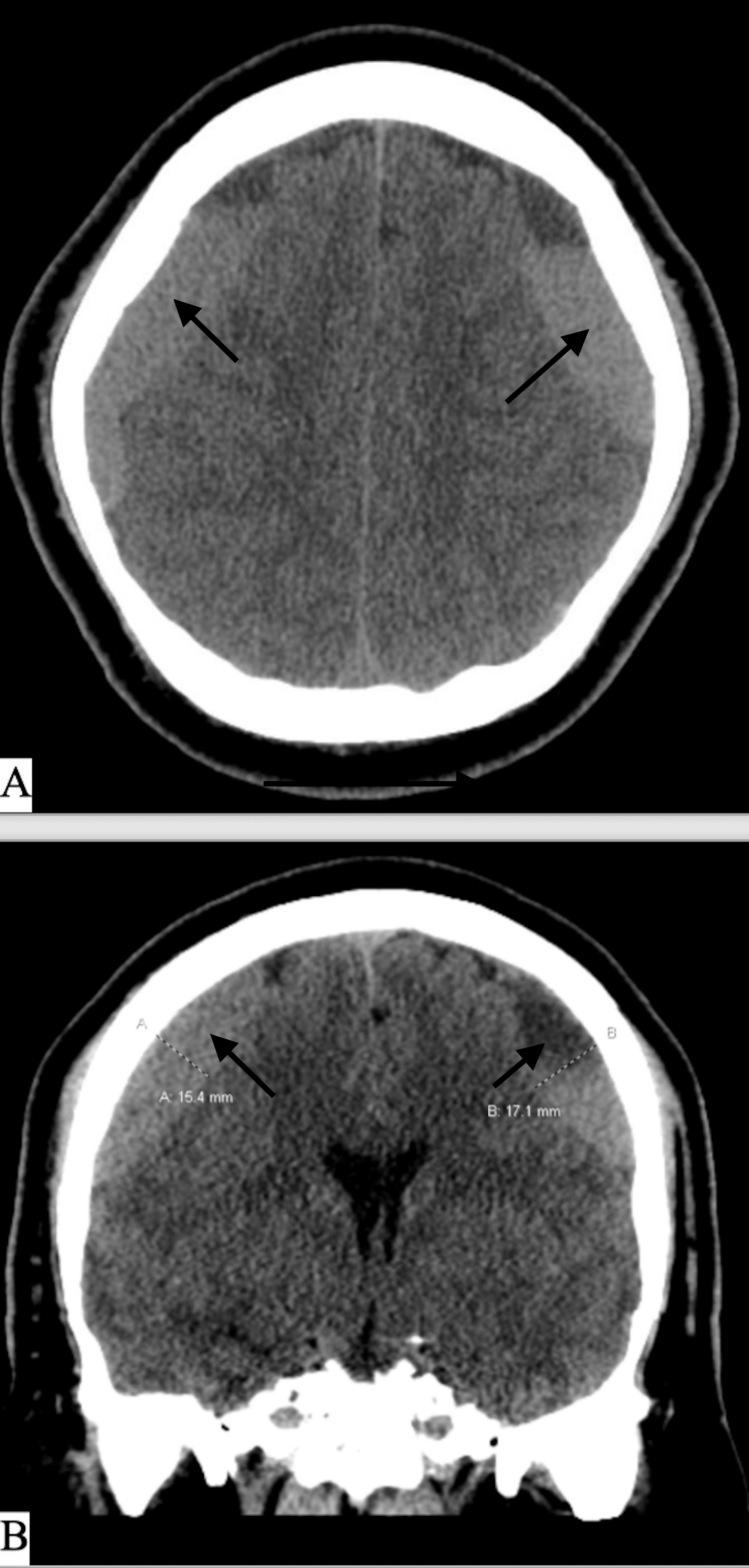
CT head on day of admission A) Axial CT head non-contrast on day of admission. B) Coronal CT head non-contrast on day of admission, showing enlargement of the bilateral subdural hematomas with mixed density components.
